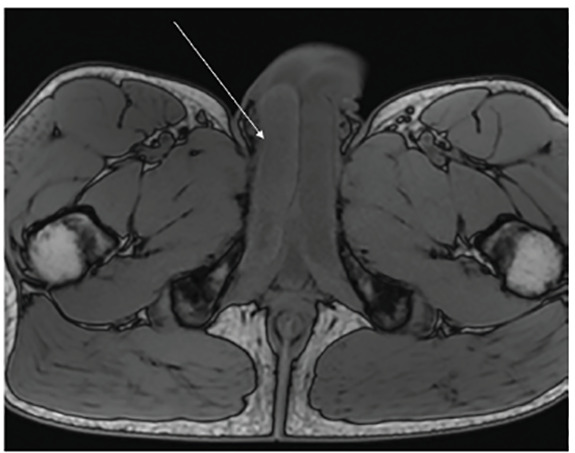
Axial T1-weighted magnetic resonance imaging of the pelvis with hyperintense signal of the right corpus cavernosum.
